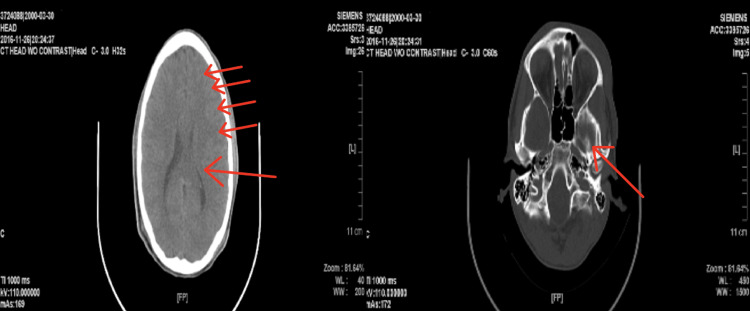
Axial CT without contrast scan of the brain was taken in 2016 (one week after the patient’s traumatic brain injury). Small red arrows show left-sided edema of the brain. A large red arrow indicates ventricular narrowing due to swelling.
